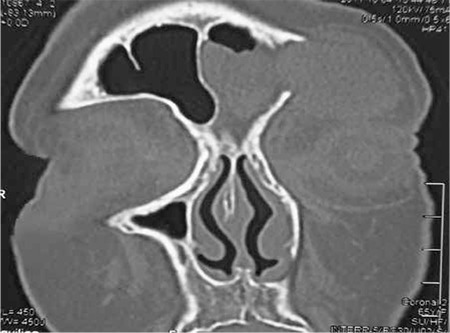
Images on paranasal sinus CT demonstrating the bony dehiscence.
